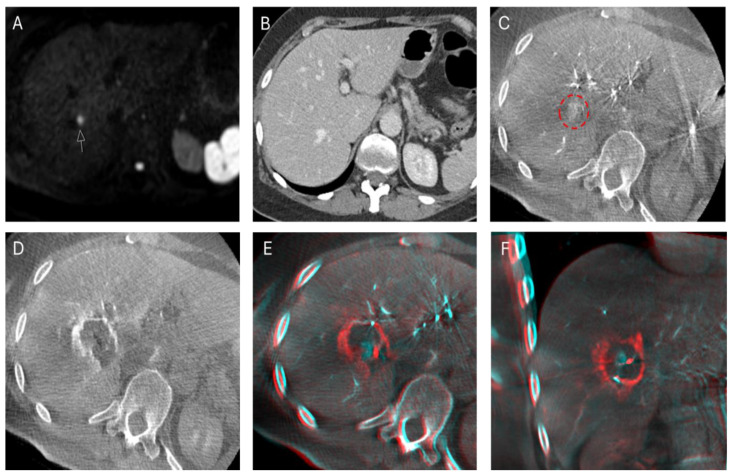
An example of an ablation of a CRLM in segment 8 using the HepACAGA technique (8 min at 120W). (A). The CRLM is visible on DWI (white arrow) (size: 9 mm); (B). The same lesion is not visible on a CT scan 28 days prior to DWI; (C). Intraprocedural detection of the lesion with C-arm-CTHA (red circle); (D). The ablation zone depicted with C-arm-CTHA immediately after ablation; (E,F). Pre- and post-ablation C-arm-CTHAs are fused using the XperGuide software in the axial and coronal planes, showing adequate ablation margins.
